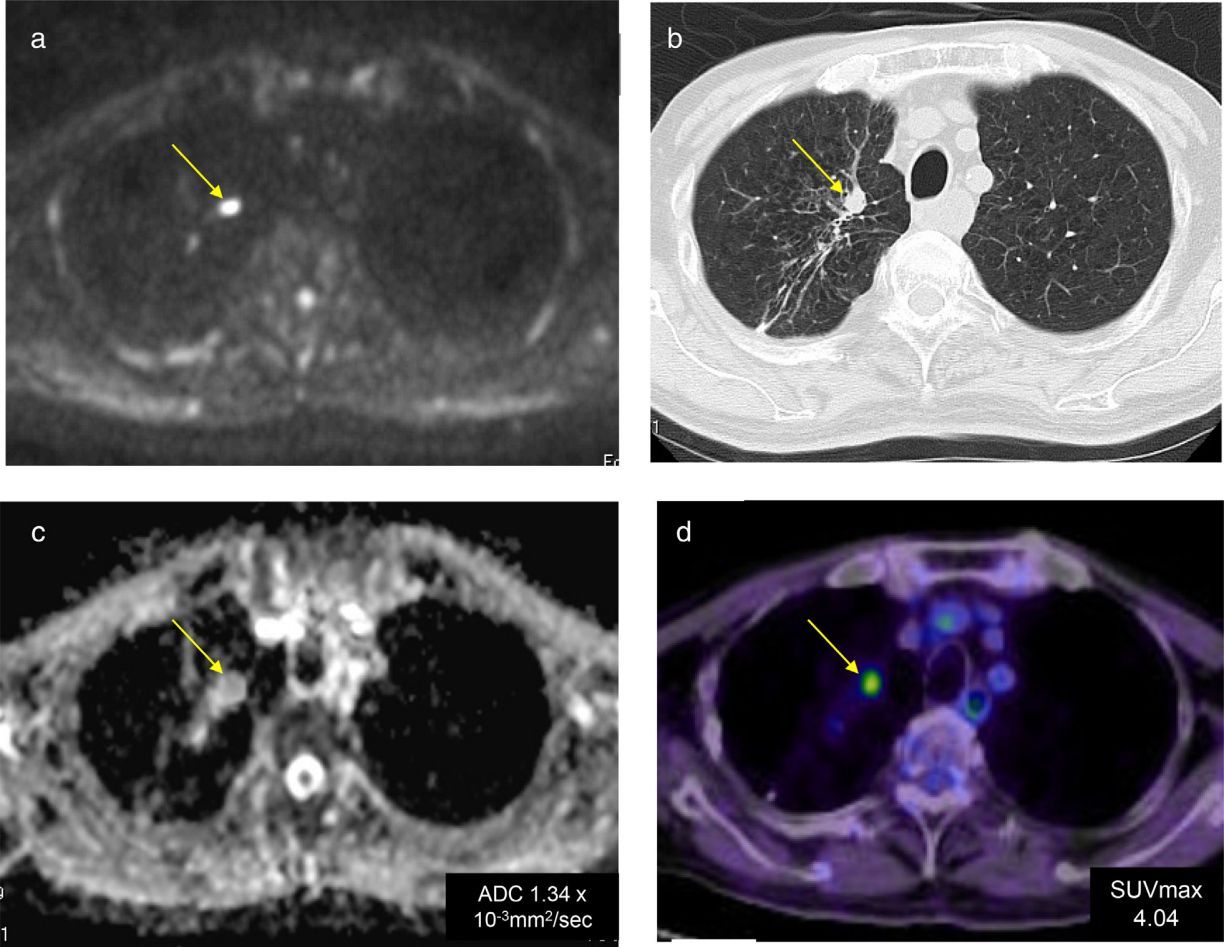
Case 2. Adenocarcinoma, pT2aN0M0. (a) Pulmonary metastasis was present on diffusion‐weighted whole‐body imaging with background suppression (DWIBS); (b) computed tomography (CT); and (d) FDG‐PET/CT. (c) The apparent diffusion coefficient (ADC) of pulmonary metastasis in ADC map was 1.34 × 10^−3^ mm^2^/s (d) The SUVmax of the pulmonary metastasis on FDG‐PET/CT was 4.04
